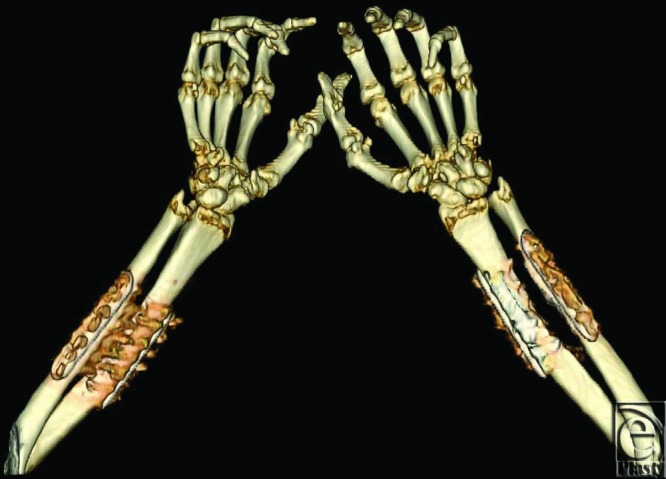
Bilateral upper extremity CT. 3D volume rendered images of both upper extremities obtained 8 months after transplantation demonstrates osseous fusion of the donor and recipient radii and ulnae in subject 2. The fingers are held in flexion at the proximal interphalangeal and distal interphalangeal joints.
